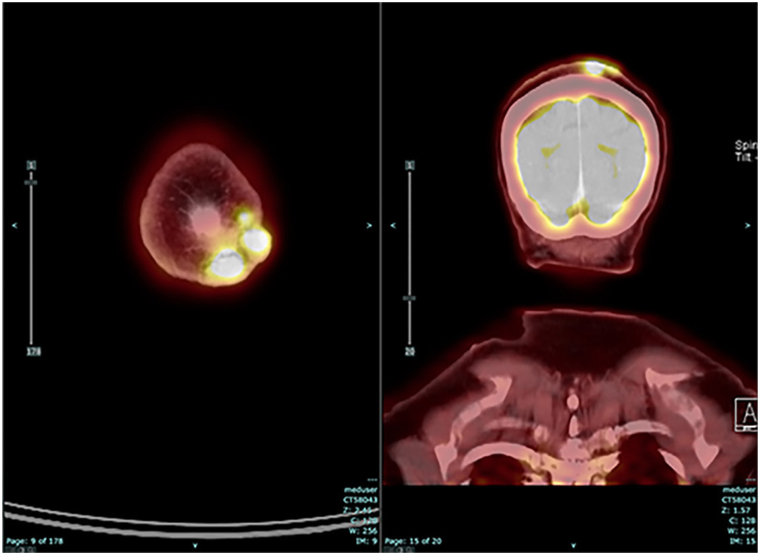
Fused 18F-fluorodeoxyglucose (FDG)-PET-CT axial and coronal images before therapy, size of scalp lesion 2.9 cm × 4.0 cm, SUV_max_ 13. *FDG*, fluorodeoxyglucose; *PET-CT*, positron emission tomography-computed tomography; *SUV*_*max*_, maximum standardized uptake value.
